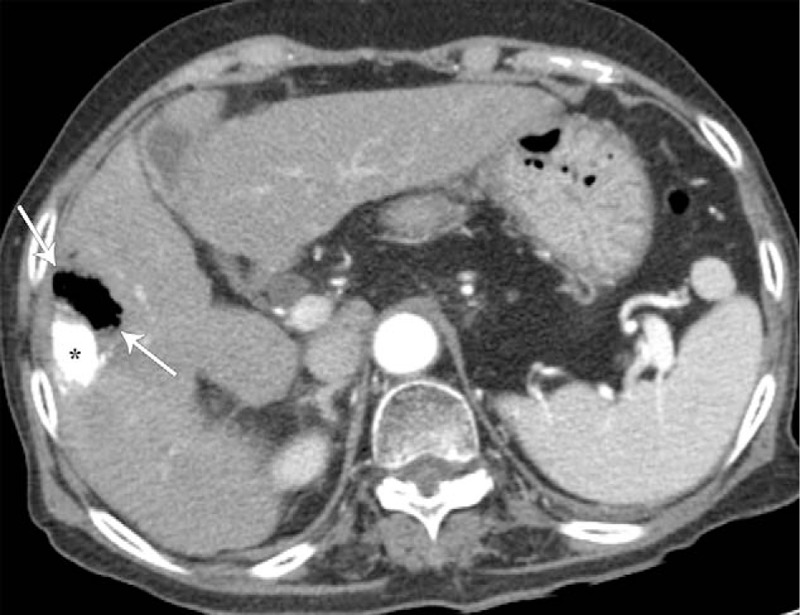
Two-week follow-up contrast-enhanced axial CT image after second TACE for a recurrent HCC shows an air-filled abscess (arrows) around a compact lipiodolized lesion (asterisk) in the right hepatic lobe. CT = computed tomography, HCC = hepatocellular carcinoma, TACE = transarterial chemoembolization.
